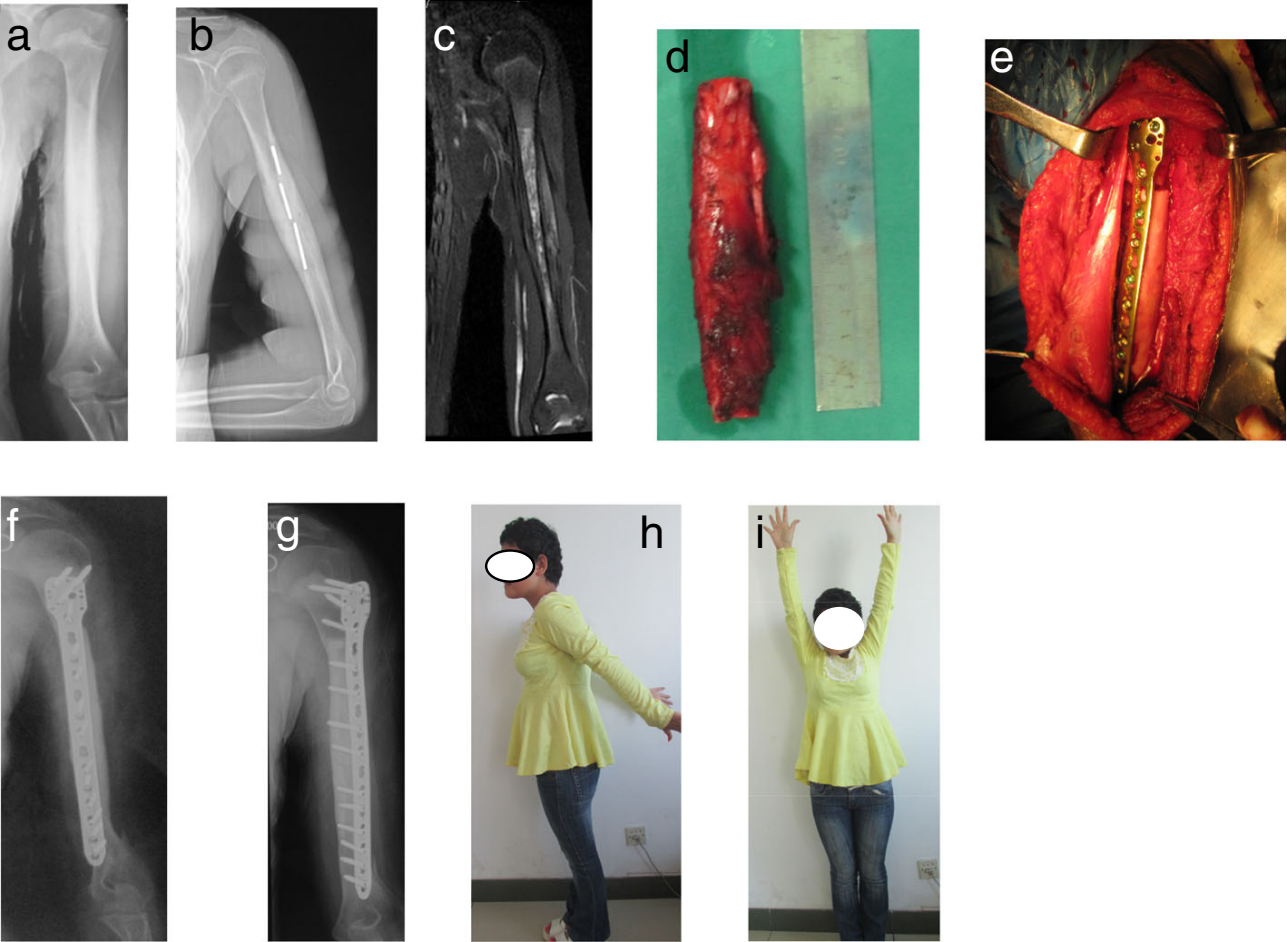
Case 3, a 11-y old female patient with Ewing sarcoma in the diaphysis of left humerus (S5). The preoperative bone strength score is 9. a, b The preoperative X-ray showed that osteogenic destruction (a) before chemotherapy and obvious calcification (b) after chemotherapy. c The preoperative MRI showed that abnormal intramedullary high signal with clear borders on T2WI. d, e This patient underwent resection of the tumor segment and replantation of the alcohol-inactivated autograft followed by plate internal fixation. f, g 5 years after operation,the X-ray indicated that internal fixation was in excellent position with no local tumor recurrence. h, i Postoperative shoulder joint function returned to normal range
